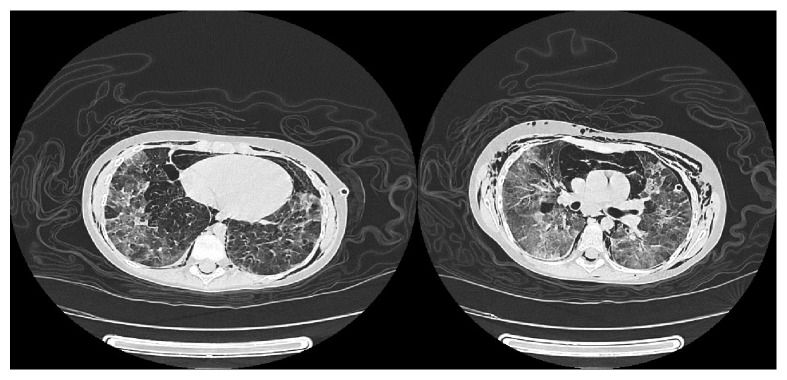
Severe emphysema in chest wall and bronchiectasis in spiral chest CT scan.
